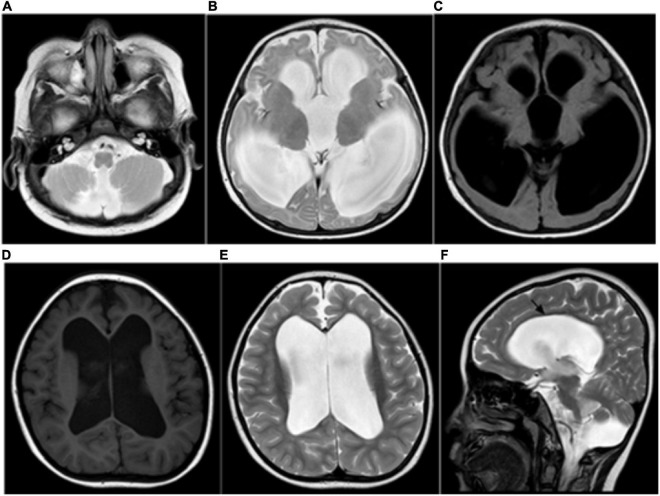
(A–C) Ventricular dilation and a small cerebellar vermis, consistent with the Dandy–Walker variant. (D–F) MRI showing severe ventricular dilation and corpus callosal thinning (black arrow, sagittal T2WI).
